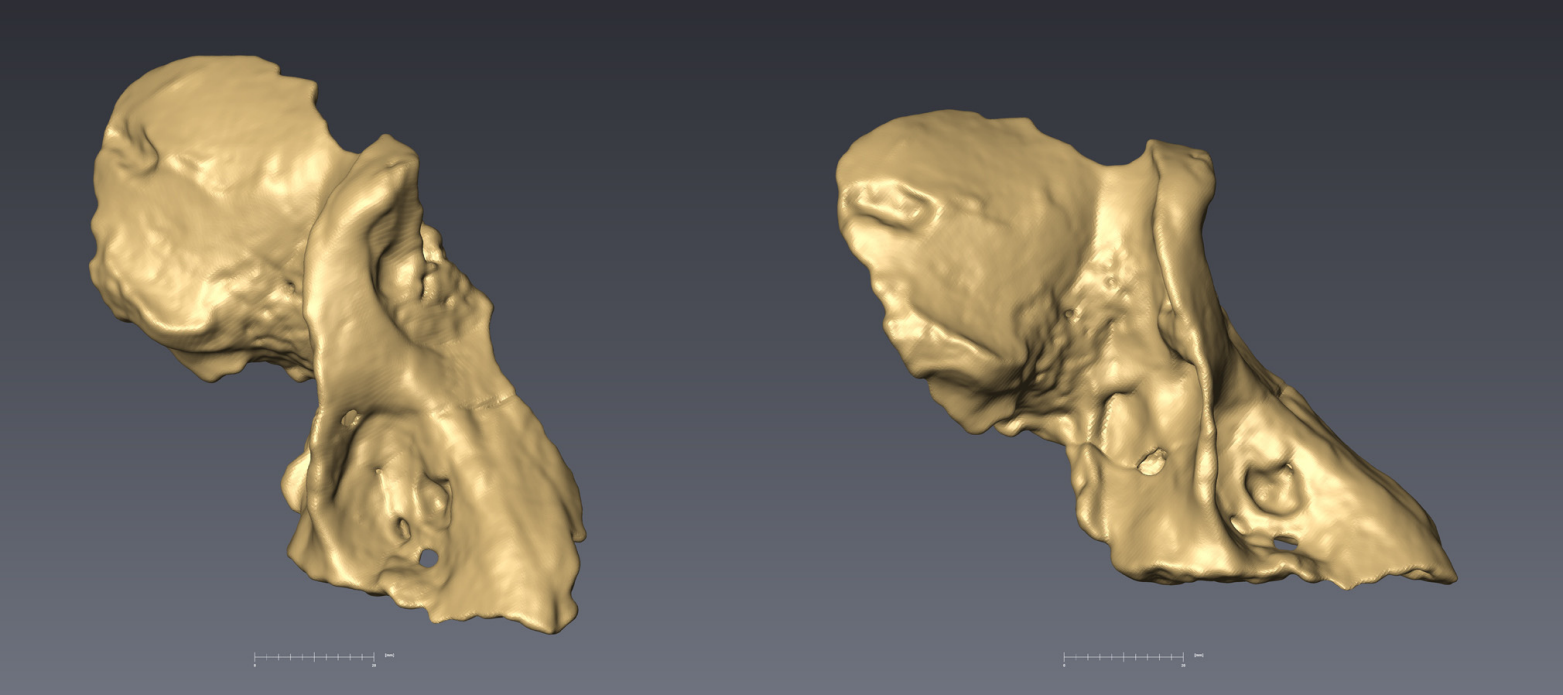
CT Scans of UW 88–866 in oblique (left) and lateral (right) views. Again, note the strong maxillary ridges, deep maxillary fossae, strong temporal lines, and tall malar region, distinctive of *P*. *angusticeps* males.
